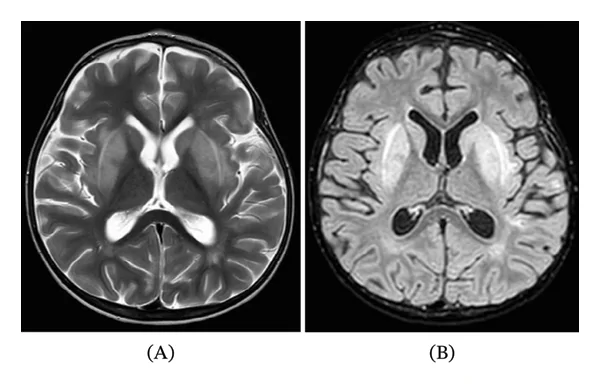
Brain MRI for the patient after 2 weeks from the initial presentation: (A) T2 weighted image at the level of the basal ganglia shows bilateral symmetric hyperintensity signal involving caudate, putamen, and external capsule. (B) FLAIR axial image at the level of basal ganglia showed the same findings.
